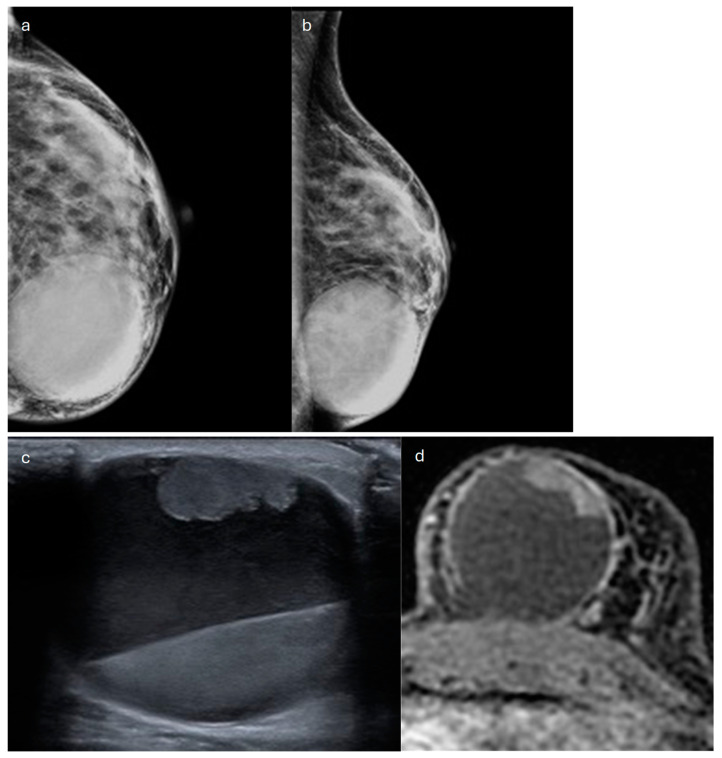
Papillary carcinoma in a 59-year-old patient. (a) Cranio-caudal and (b) medio-lateral oblique mammograms of the left breast show a round opacity in the lower-inner quadrant (c) corresponding on ultrasound to a complex cystic mass with a solid anterior component. A fluid−fluid level is visible inside the cyst due to the bleeding produced during the biopsy procedure. (d) Axial 3D gradient echo T1-weighted post-contrast MRI image of the left breast shows the enhancement of the solid component of the lesion.
